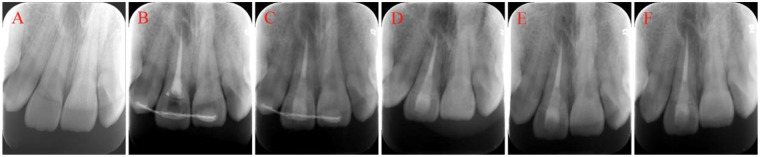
Serial periapical radiographs of tooth #11 demonstrating the treatment sequence: (A) initial presentation of the crown-root fracture; (B) post-obturation radiograph confirming adequate root canal filling; (C) radiograph after fiber post placement demonstrating coronal stabilization; (D) 3-month follow-up; (E) 6-month follow-up; (F) 12-month follow-up.
